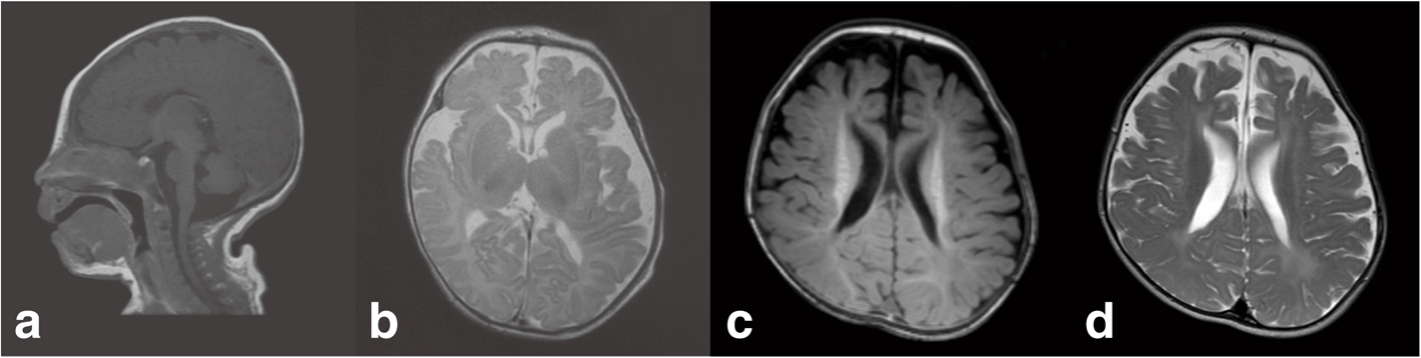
Head MRI of the siblings. a, b Head MRI of the sister at 25 days old showed skull deformity and asymmetrical bilateral structure. c, d Bilateral ventricle enlargement, asymmetry of brain structure, decreased white matter and thin corpus callosum of the proband at 8 months old
